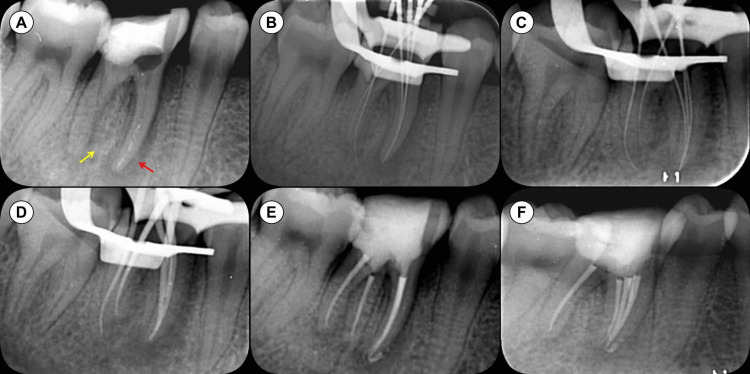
Periapical radiographs taken during root canal procedures for tooth #46 (A) The pre-operative radiograph of tooth #46 reveals previous treatment and significant structural reduction, along with a “J” shaped periapical lesion around the mesial root (indicated by a red arrow). There is radiopaque material in the mesial canals. Additionally, the tooth features an extra distolingual root known as radix entomolaris (yellow arrow). (B,C) Periapical radiographs captured from two different angles to ascertain the working length. (D) A periapical radiograph during the fitting of the master gutta-percha cones. (E,F) Post-obturation periapical radiographs, each taken from a different angle.
